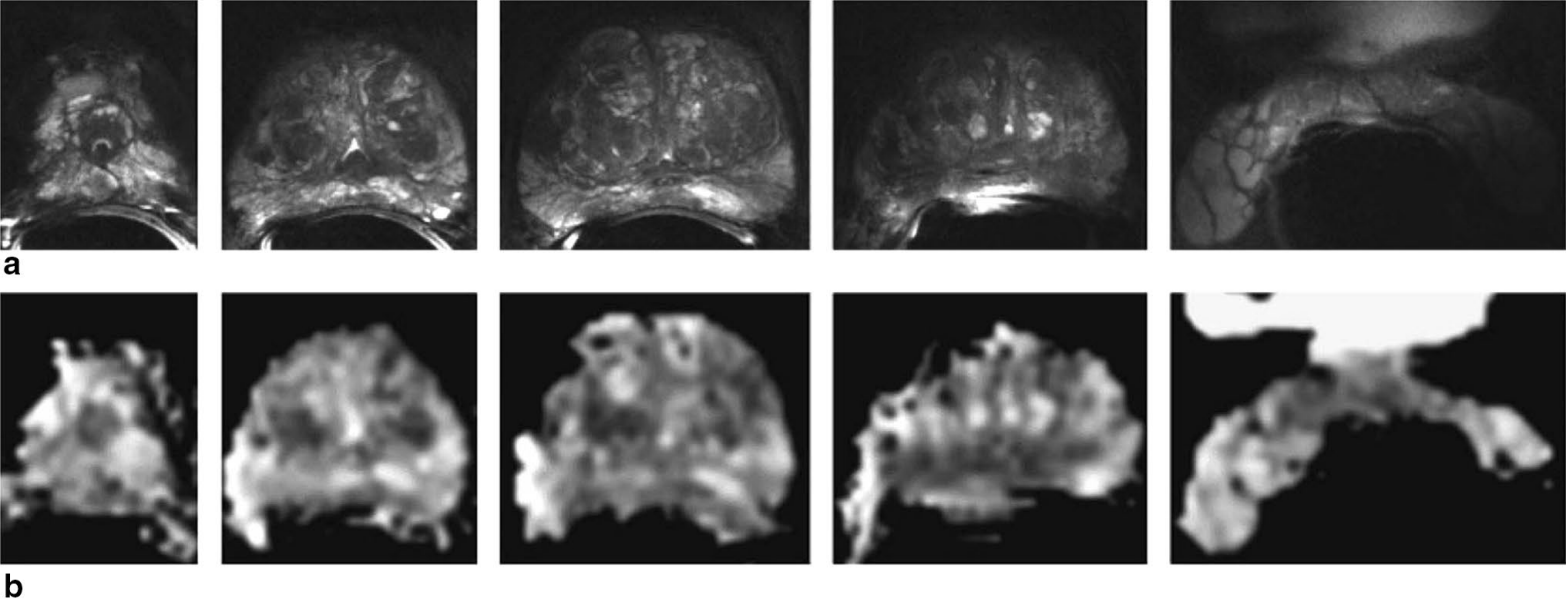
MRI with an ERC at 7 T of the prostate of a 62-year-old patient with prostate cancer (Gleason score 3 + 3). a Five transversal T_2_w turbo spin-echo images from a series of 23 slices from apex to seminal vesicles, resolution 0.3 × 0.3 × 2.0 mm^3^. b Transversal ADC maps at corresponding locations (5 images from 23 slices), resolution 1.75 × 1.75 × 2.0 mm^3^. Reprinted with permission from Lagemaat et al. [41]
